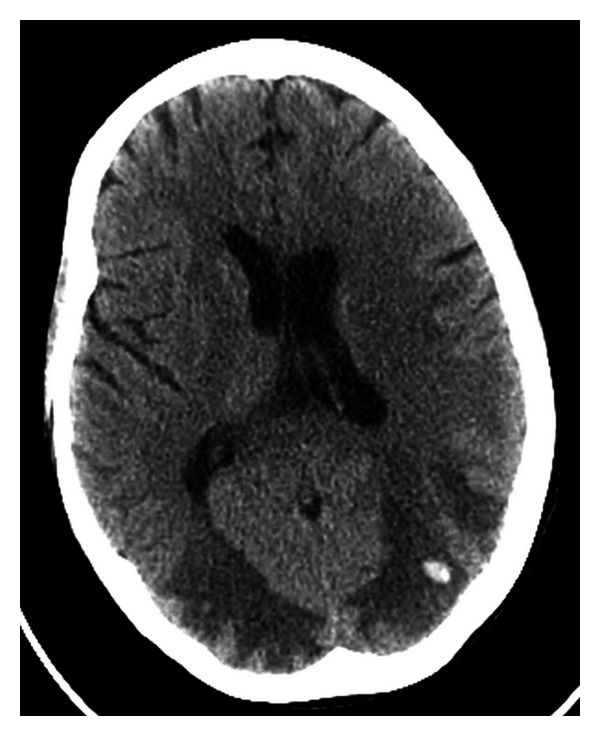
CT without contrast, hypodensity noted in the posterior parietal-occipital regions bilaterally and a small hemorrhage in the left parietooccipital region.
